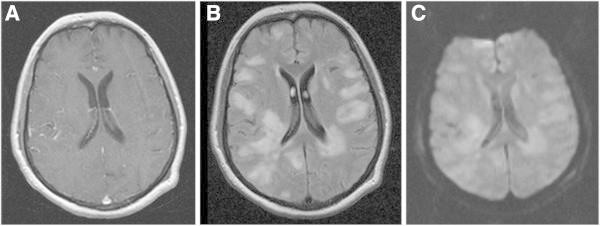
MRI-scan 10 days after symptom onset. Progressive widespread white matter lesions with restriction in diffusion but still unaffected blood-brain-barrier. A) T1 Gd-enhanced, B) T2-FLAIR, C) DWI.
